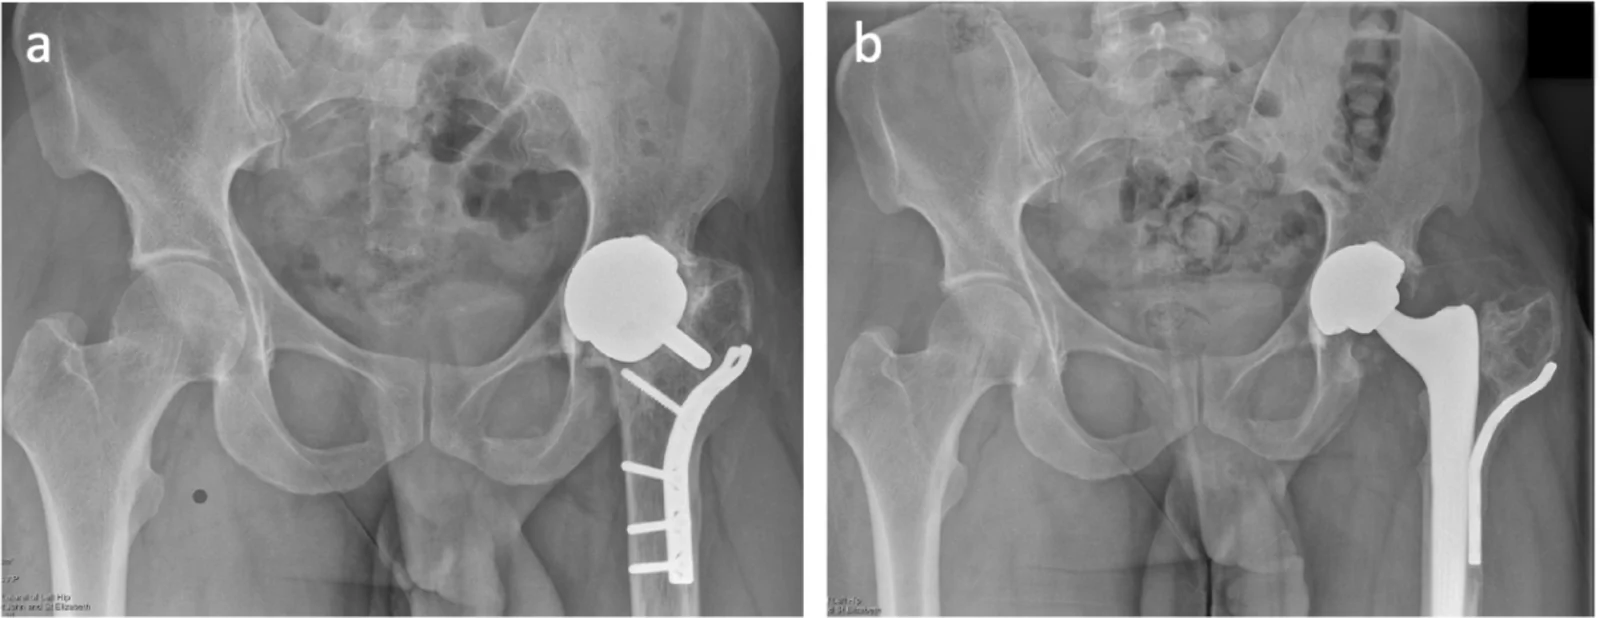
Anteroposterior pelvis radiographs of a failed hip resurfacing due to secondary avascular necrosis. a Preoperative radiograph showing the failed construct. b Postoperative radiograph following conversion to a total hip arthroplasty with retention of the well-fixed MoM acetabular shell
